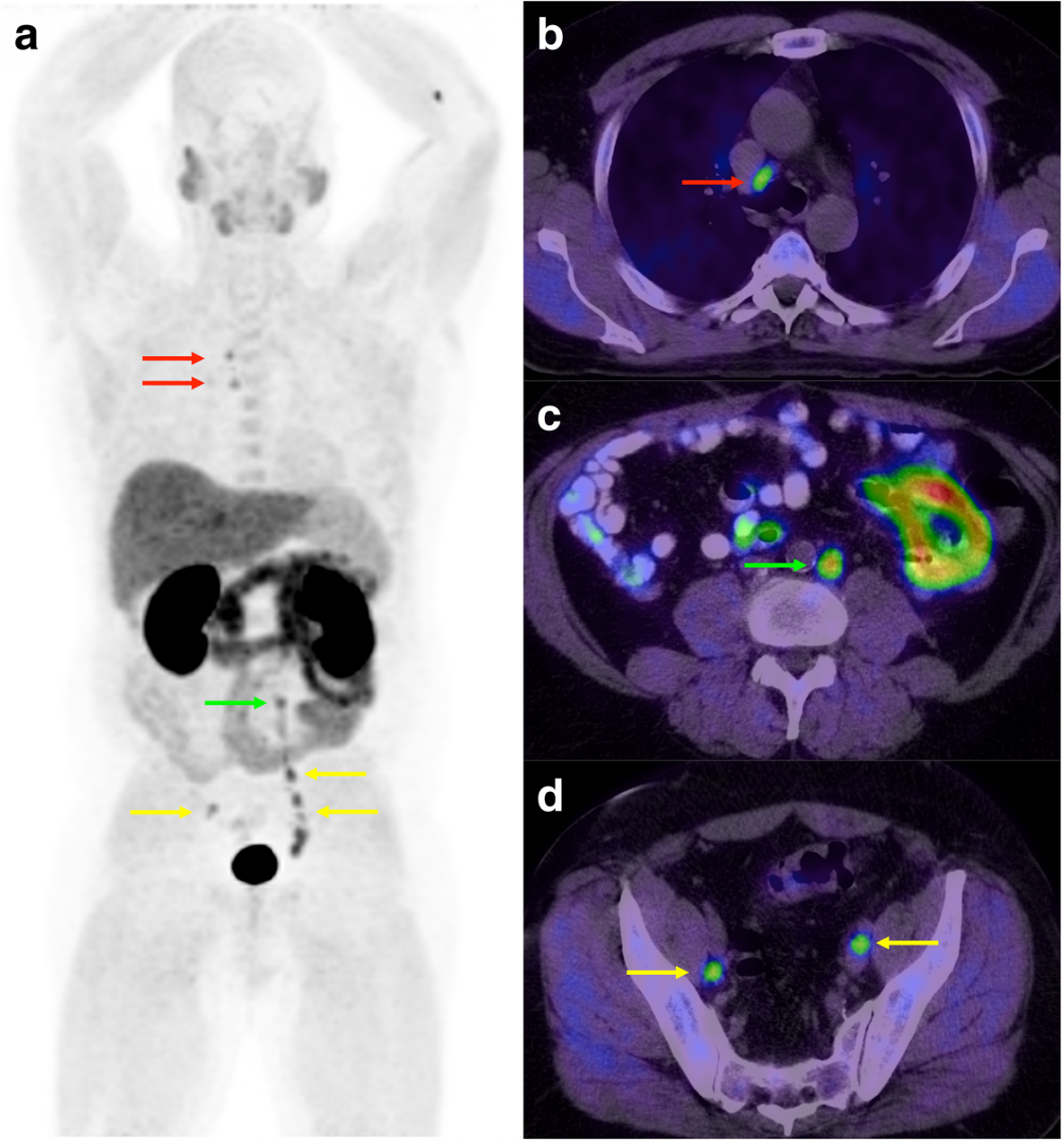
Restaging FCH-PET/CT in a 58 y.o. patient with biochemical relapse following external beam radiotherapy. Gleason sum was 9 on initial biopsy. PSA at time of examination was 29.3 mg/L. Whole-body MIP (a) and transaxial fusion series (b, c, d) showed intense uptake in metastatic lymph nodes in the right paratracheal (red arrows), left para-aortic (green arrows) and bilateral iliac areas (yellow arrows)
